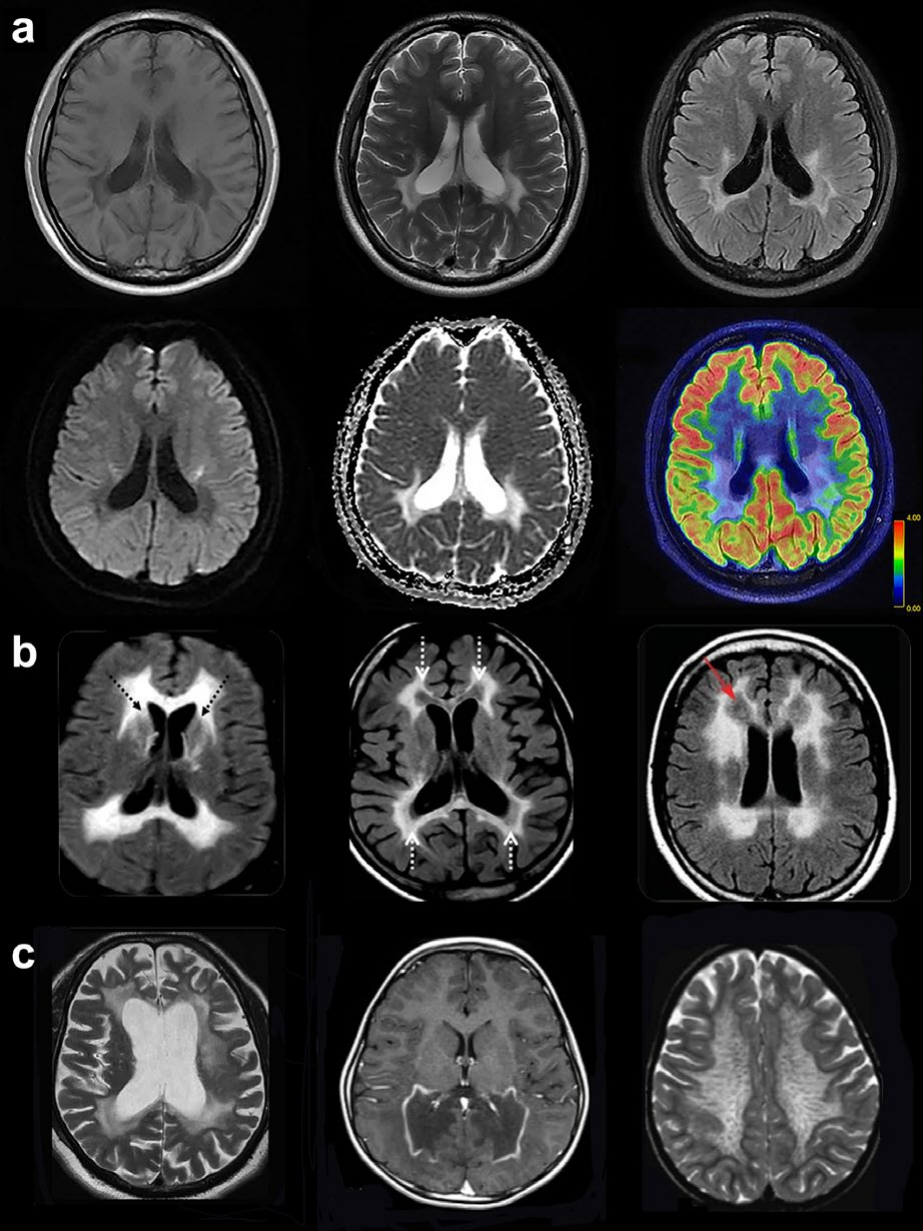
Brain MRI scans of patients with various types of leukoencephalopathy. (a) Brain MRI of P20 (the presented case) with AARS2‐related leukoencephalopathy. T1‐weighted, T2‐weighted, axial scans show symmetrical and extended white matter abnormalities at bilateral periventricular areas and corpus callosum involvement mainly in the posterior part. Fluid‐attenuated inversion recovery (FLAIR)‐weighted, axial scans present symmetrical, longitudinal, striped, and intense signals present on both sides of the corticothalamic level. The diffusion‐weighted image (DWI) and apparent diffusion coefficient (ADC) image show patchy areas of restricted diffusion in the abnormal white matter. PET MRI of the patient shows that decreased metabolism is pronounced in the white matter lesions. (b) T2‐weighted, axial scans of Patient 3 at age 33 years (left) demonstrates pronounced leukoencephalopathy at bilateral periventricular areas. Axial T2‐weighted MRI of Patient 4 at age 24 years (middle) shows that abnormal signals are found in the frontal and parietal lobe white matter and the pyramidal tract at the internal capsule. FLAIR MRI image of Patient 7 at age 30 (right) shows that the affected white matter is rarefied. (c) In a patient with ALSP (left), axial T2‐weighted MRI shows confluent slightly asymmetric frontoparietal‐predominant white matter hyperintensity, with more atrophy compared with AARS2 leukoencephalopathy. In a patient with X‐ALD (middle), axial postgadolinium contrast sequences show diffuse white matter signal T2‐weighted hyperintensity and volume loss of the posterior frontal, posterior callosal and parieto‐occipital regions. In a patient with MLD (right), an axial T2‐weighted sequence demonstrates widespread symmetrical white matter signal hyperintensity sparing the subcortical U fibers and radiating transmedullary bands
